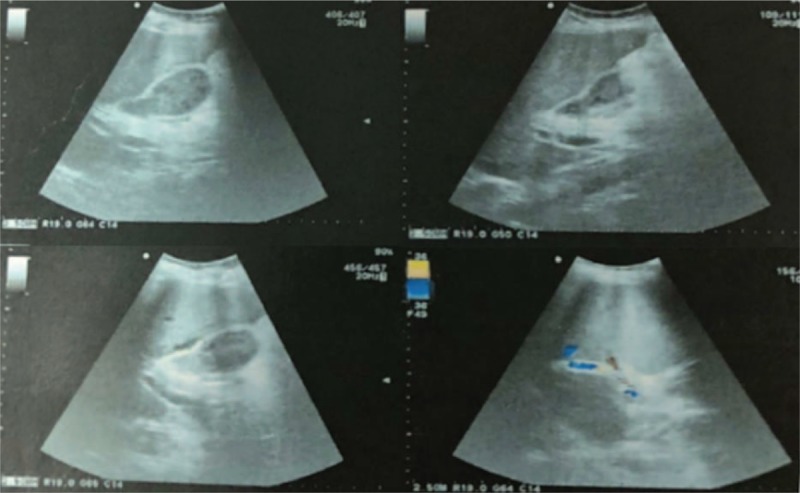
Figures of ultrasound after TIPS. There is moderately strong echo filling in the gallbladder and CBD. CBD = common bile duct, TIPS = transjugular intrahepatic portosystemic shunt.
